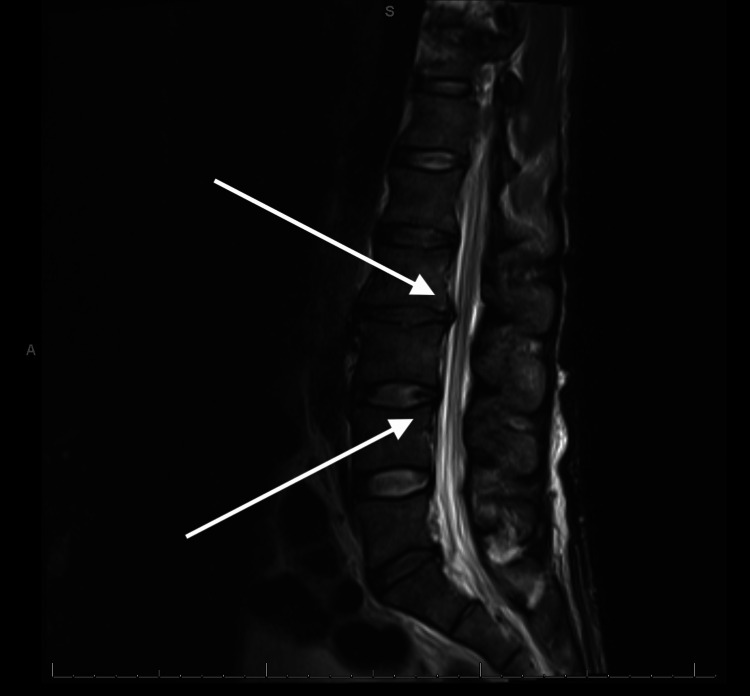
MRI of the lumbar spine showing compression of the spinal cord and spinal canal stenosis
